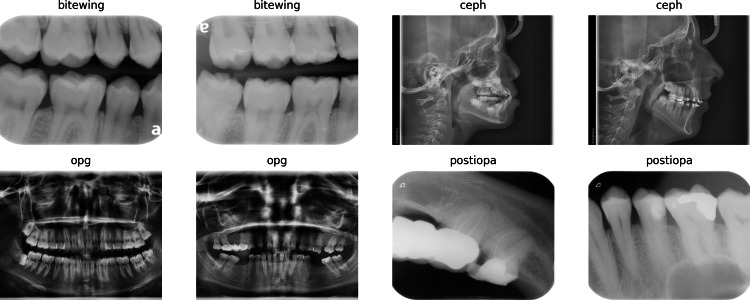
Samples of radiographs used for training, validation, and testing
